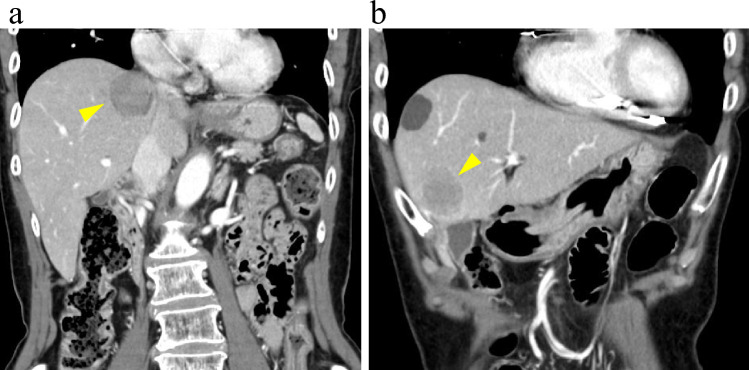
Dynamic computed tomography (CT) images at the arterial phase upon referral to our hospital. a A coronal image showing a low-attenuation area in segment 7 of the right hepatic lobe (arrowhead). b An axial image showing a low-attenuation area in segment 5 of the right hepatic lobe (arrowhead)
